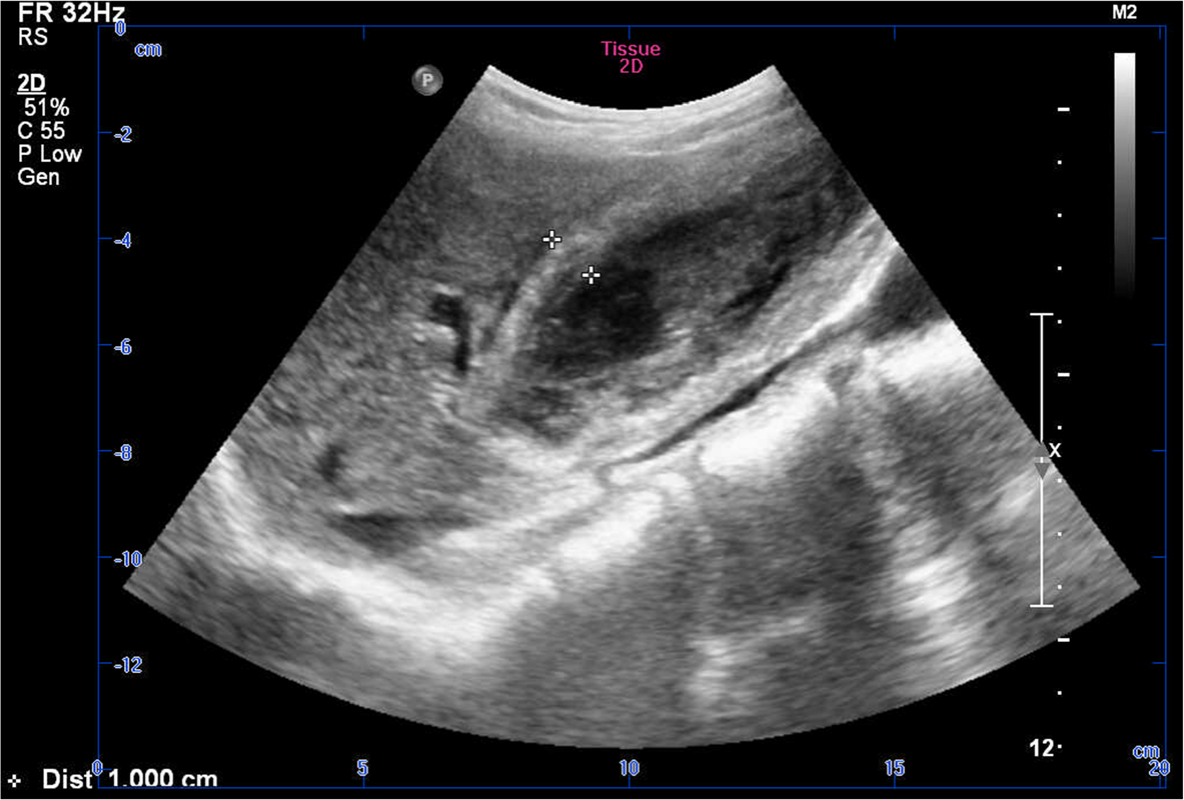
Preoperative ultrasound result indicating the thickened gallbladder wall and hyperechoic structure inside the gallbladder
